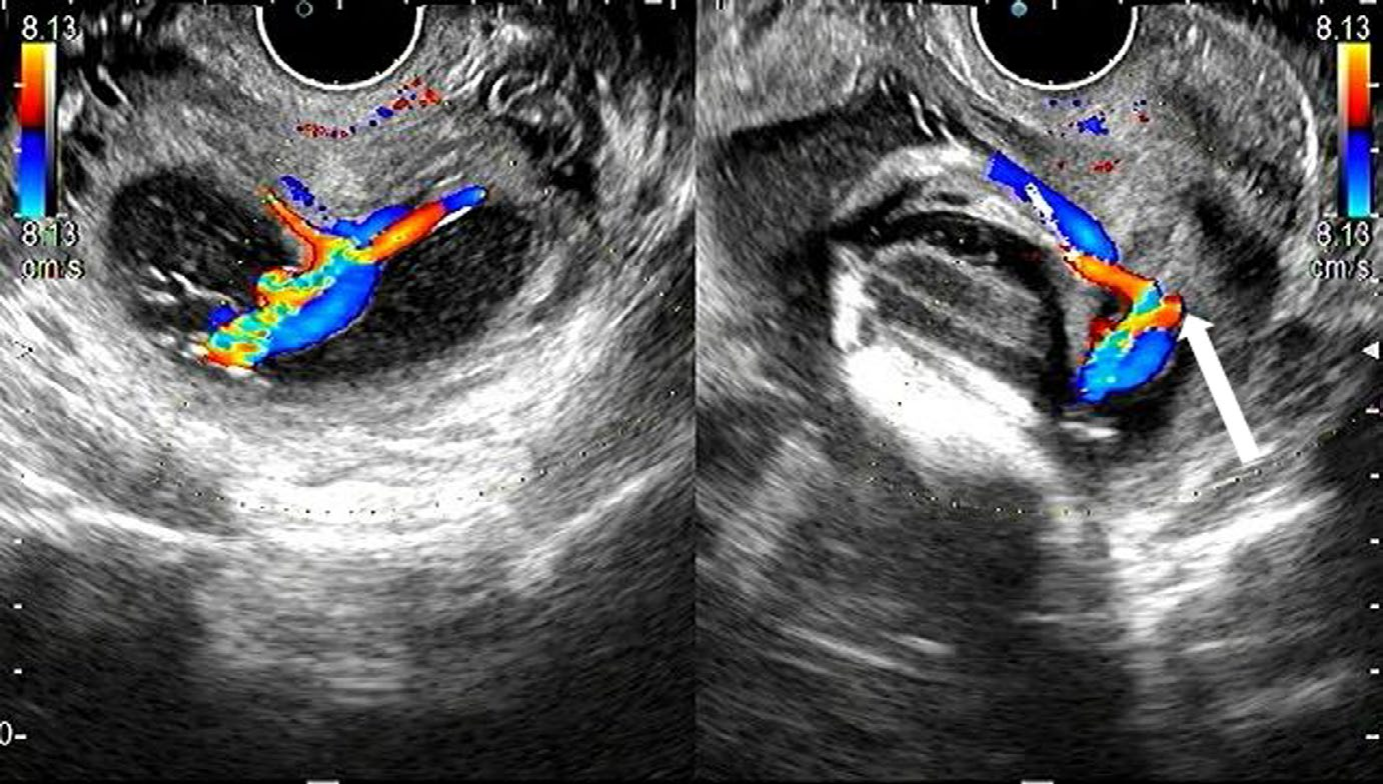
Transvaginal ultrasonography image taken at the first visit showing the vasa previa. The cord vessels are shown running on the anterior lower uterine segment and covering the internal cervical os (white arrow)
